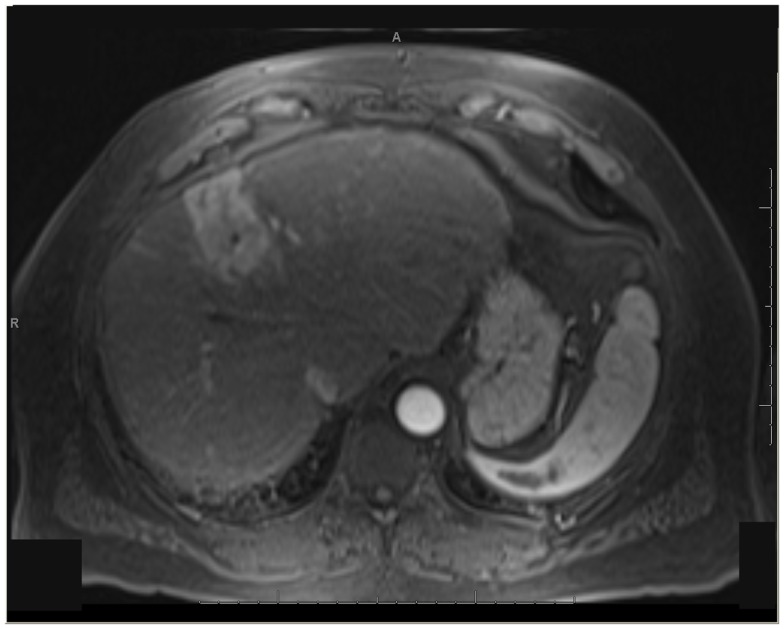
T1 MRI with early phase contrast. Pre-radioembolization.
